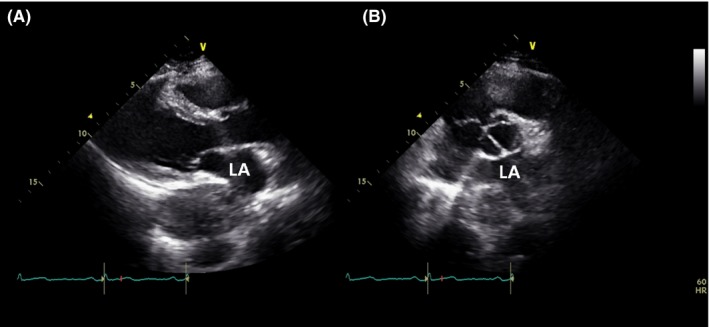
Transthoracic echocardiography revealed well‐defined heterogeneous oval mass compressing left atrium in the parasternal long (A) and short axis view (B). LA, left atrium
